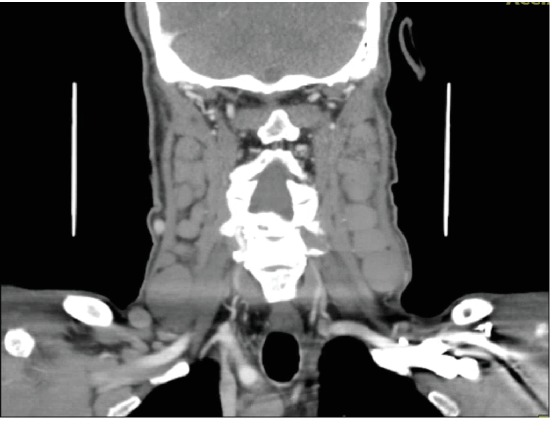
CT of the soft tissue in the neck showing diffuse cervical lymphadenopathy.
